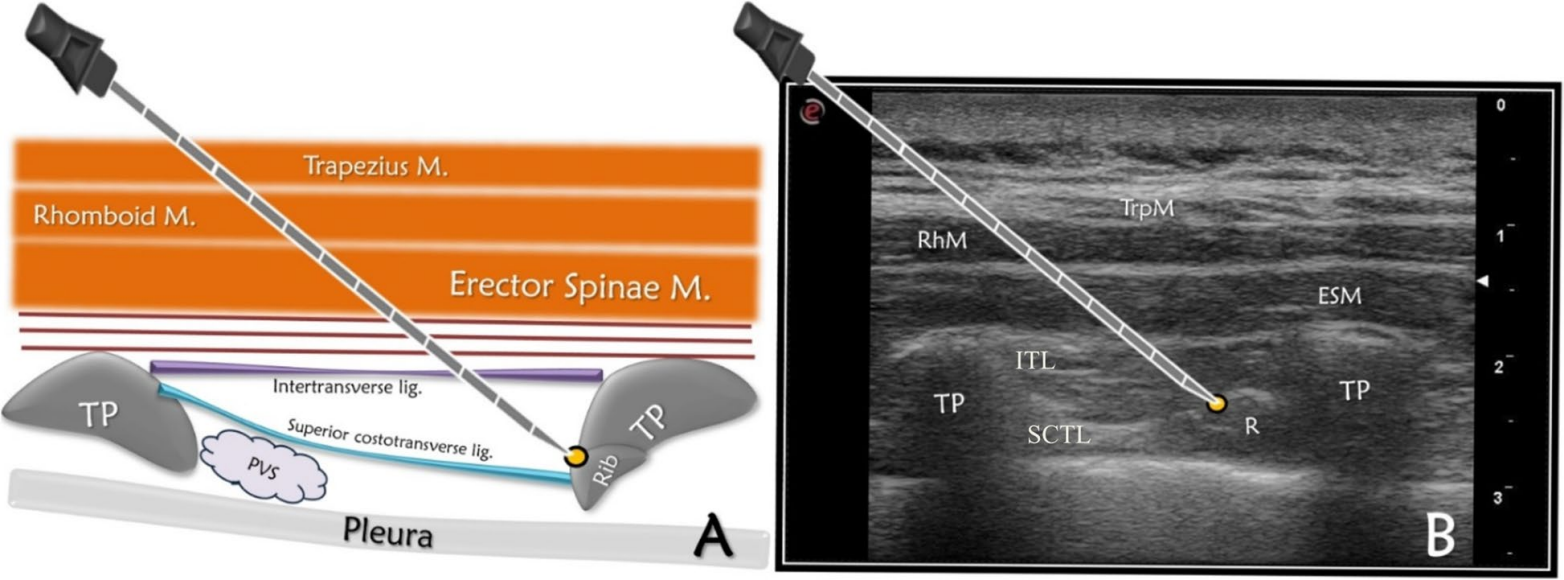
Ultrasound anatomy of costotransverse block
